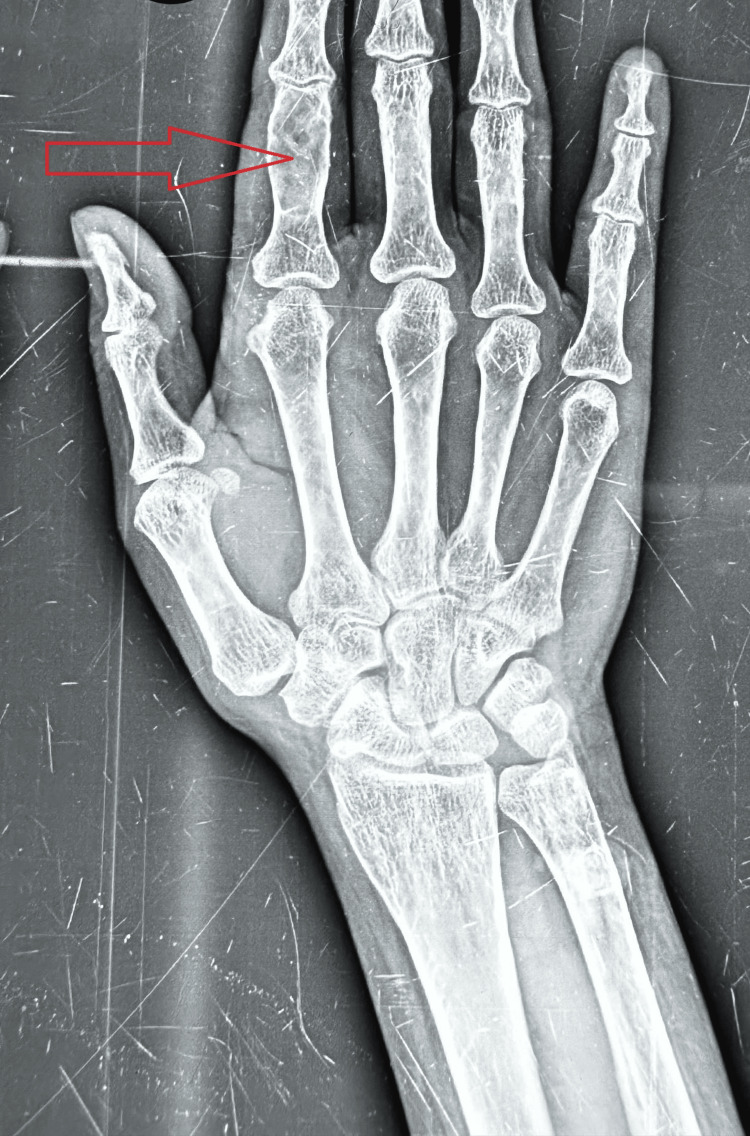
X-ray showing subperiosteal bone resorption and bone cyst in the right index finger
